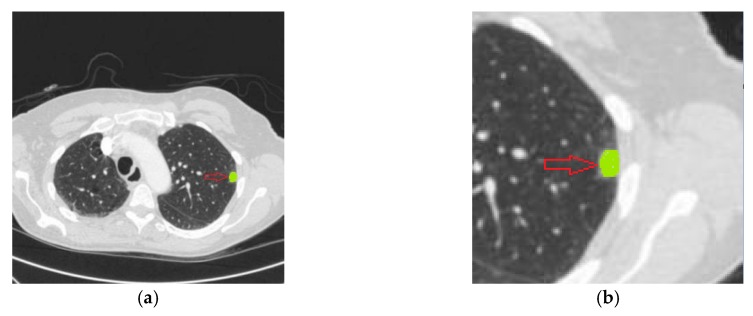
Lung CT image [13]: (a) Original image with nodule (green color), (b) the part of CT image with nodule > 3 mm ROI (green color). Note that the xml file includes the outline of the node (only its boundary points). In this case, the entire nodule is displayed for better understanding and visibility.
